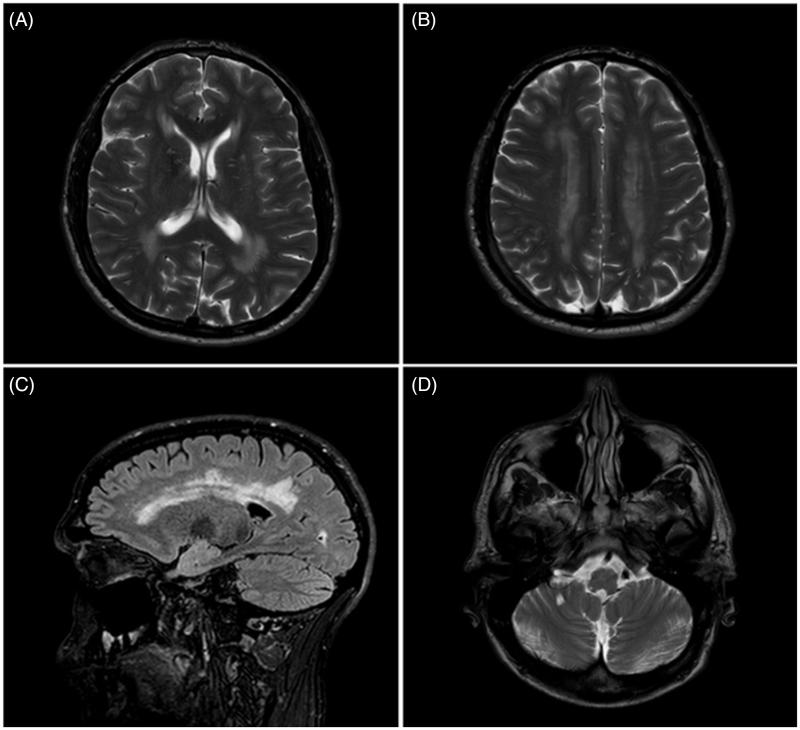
MRI brain scan from the patient with concurrent Fabry’s disease and amyotrophic lateral sclerosis. (A and B) T2 weighted transverse MRI scan showing symmetrical white matter change; (C) Sagittal FLAIR MRI scan showing white matter changes in the cerebral hemispheres; and (D) T2 weighted transverse MRI showing white matter changes in the brainstem.
